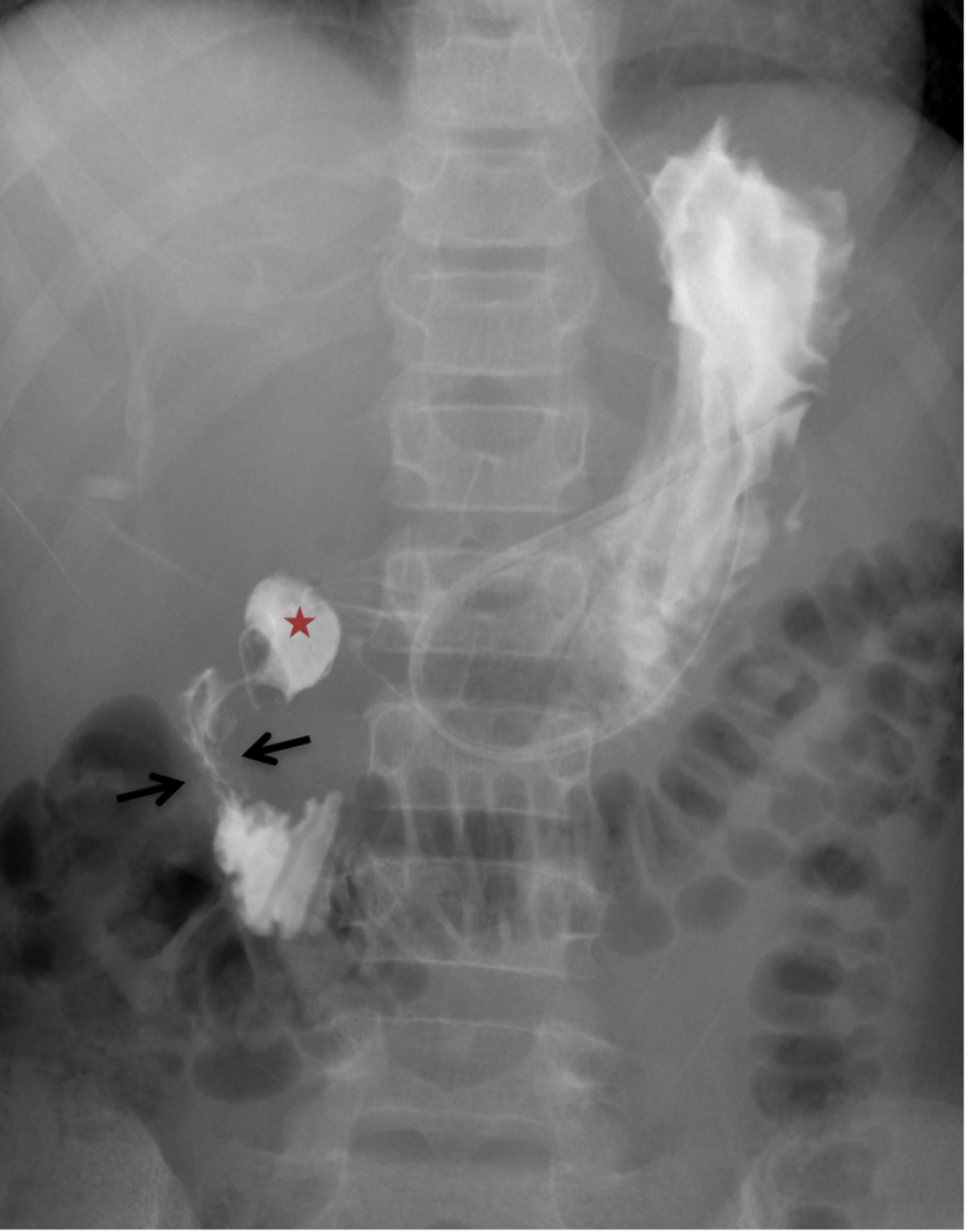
Selected frontal radiograph from gastrografin swallow study demonstrates the contrast filled out-pouch from the second part of the duodenum (star). Note the narrowing of the duodenal lumen with thumb printing (arrows) changes suggestive of bowel wall edema.
